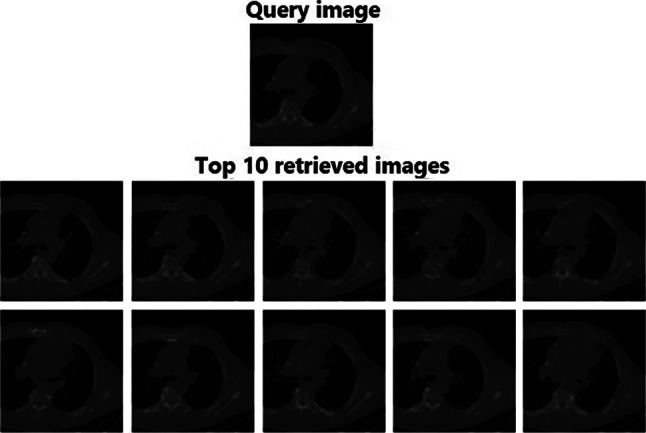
Retrieved images for a NEMA-CT database query using RbQE with VGG-19
